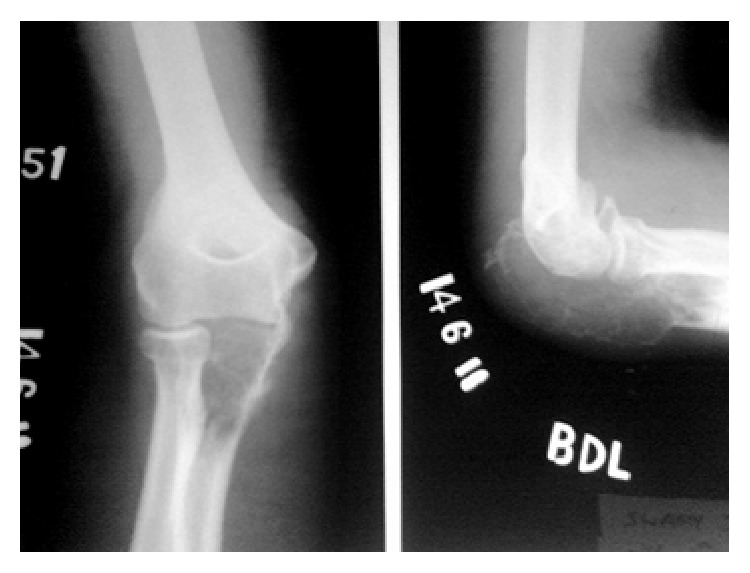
X-ray at the time of presentation.
